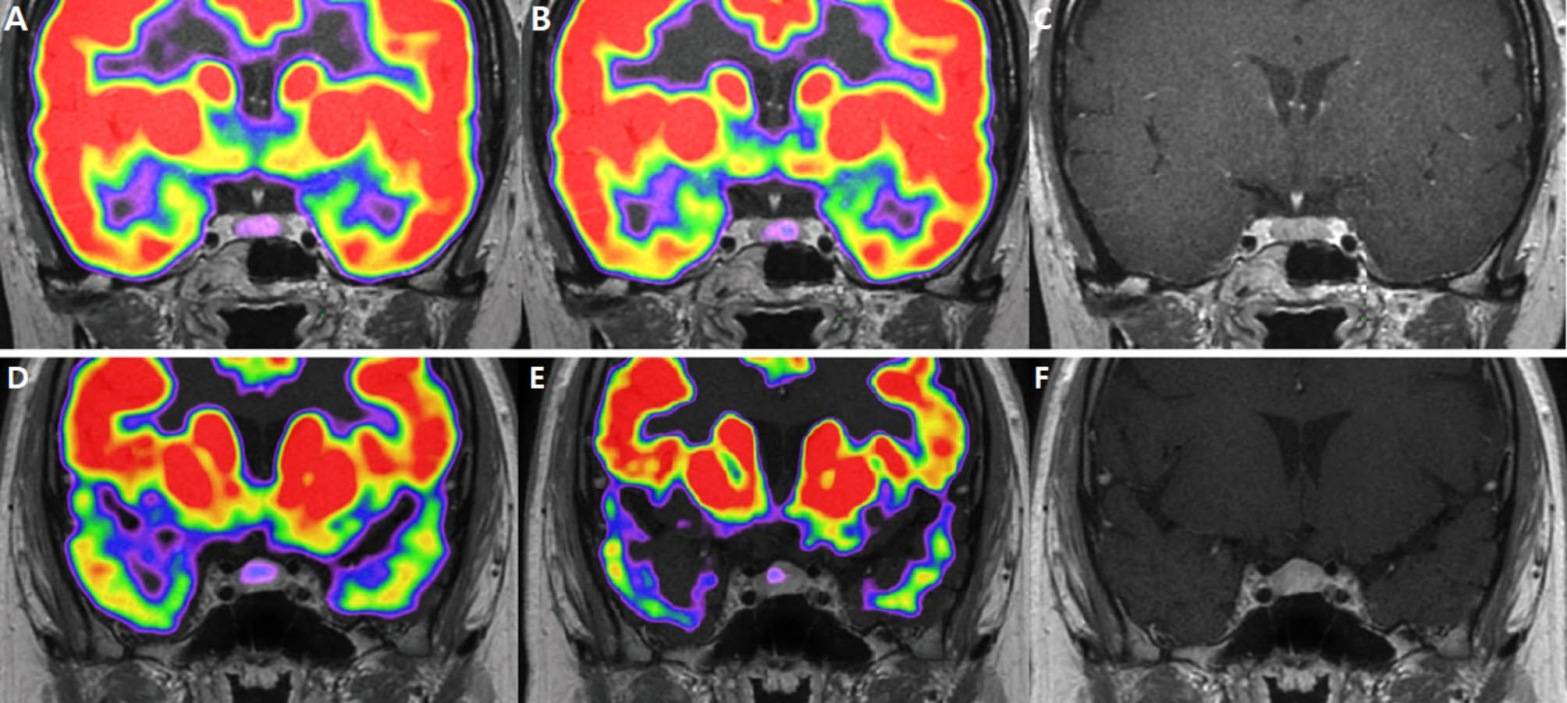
Images for corticotroph adenomas that appear different for localization in^18^F-FDG PET/CT. 9 mm sized adenoma in the left lateral wing of pituitary gland. It was found in the left lateral wing of the pituitary gland, showing an ^18^F-FDG uptake in the pituitary fossa with an SUV_mean_ of 1.04 and SUV_max_ of 1.07. However, after DEX suppression, the left side of the pituitary gland did not exhibit suppressed ^18^F-FDG uptake, with SUV_mean_ 1.05 SUV_max_ 1.14 (A). Co-registered baseline ^18^F-FDG PET/CT and MRI images. (B). Co-registered ^18^F-FDG PET/CT and MRI images after DEX suppression. (C). MRI image 2 mm pituitary adenoma was detected at the left lateral wing, showing diffuse FDG uptake in the pituitary fossa with an SUV_mean_ of 0.86 and SUV_max_ of 1.04. After DEX suppression, focal FDG uptake was observed, with SUV_mean_ 0.87 and SUV_max_ 0.98. (D). Co-registered baseline ^18^F-FDG PET/CT and MRI images. (E). Co-registered ^18^F-FDG PET/CT and MRI images after DEX suppression. (F). MRI image
